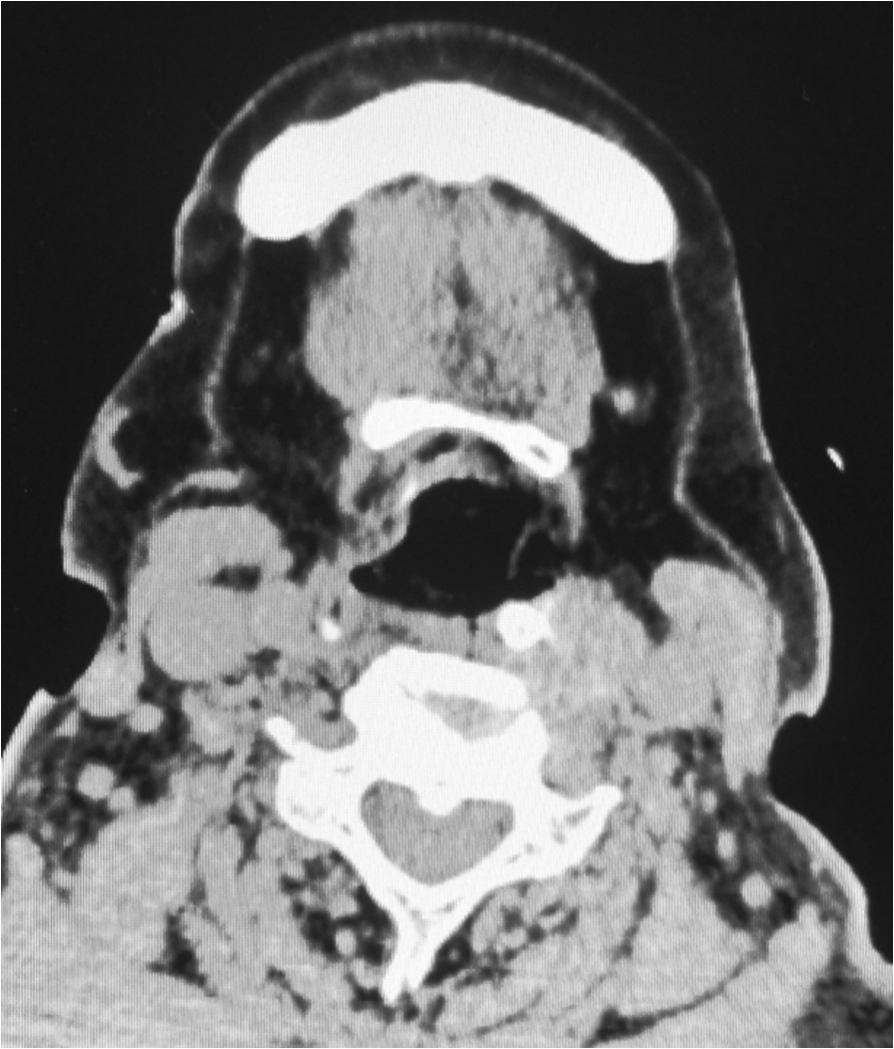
Postoperative computed tomography scans. The retropharyngeal hematoma was no longer seen.
